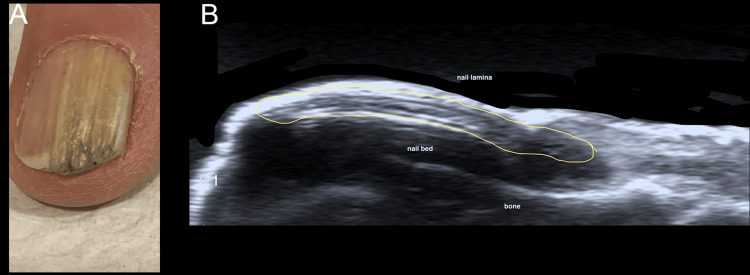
Clinical and ultrasound features of onychomatricoma A: clinical image of the left index showing acquired localized longitudinal xantopachyonychia (band pattern of nail plate thickening with yellow discoloration); B: longitudinal greyscale ultrasound image of the nail delineated by a yellow line showing solid mass, originating above matrix zone and extending along the nail plate with multiple parallel horizontal hyperechogenic lines, characteristic of onychomatricoma. Original unpublished image, courtesy of Dr. Claudia Gonzalez.
